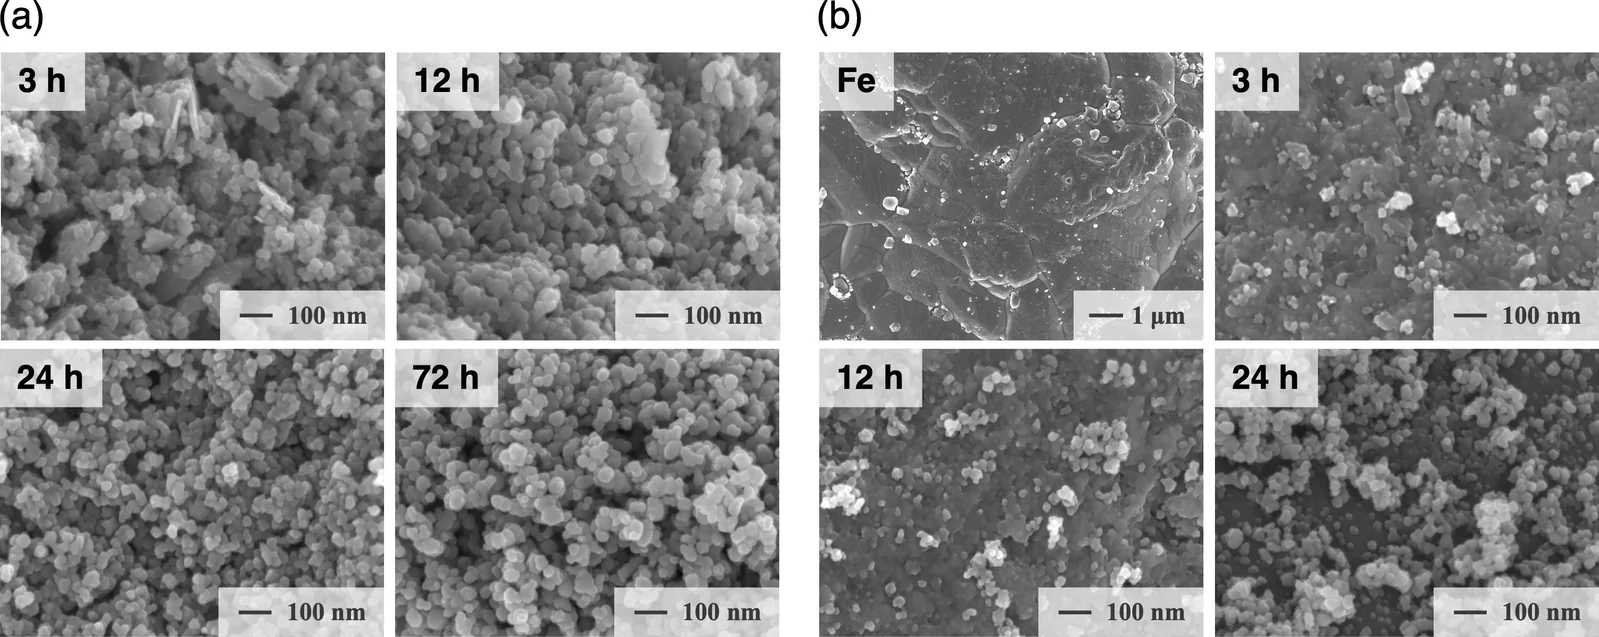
SEM images of samples obtained by ultrasonic irradiation of Fe powder in water at 23 kHz and 40 °C: (a) powders recovered from the supernatant after 3, 12, 24, and 72 h of irradiation and (b) starting Fe powder and residual solids recovered after 3, 12, and 24 h of irradiation.
